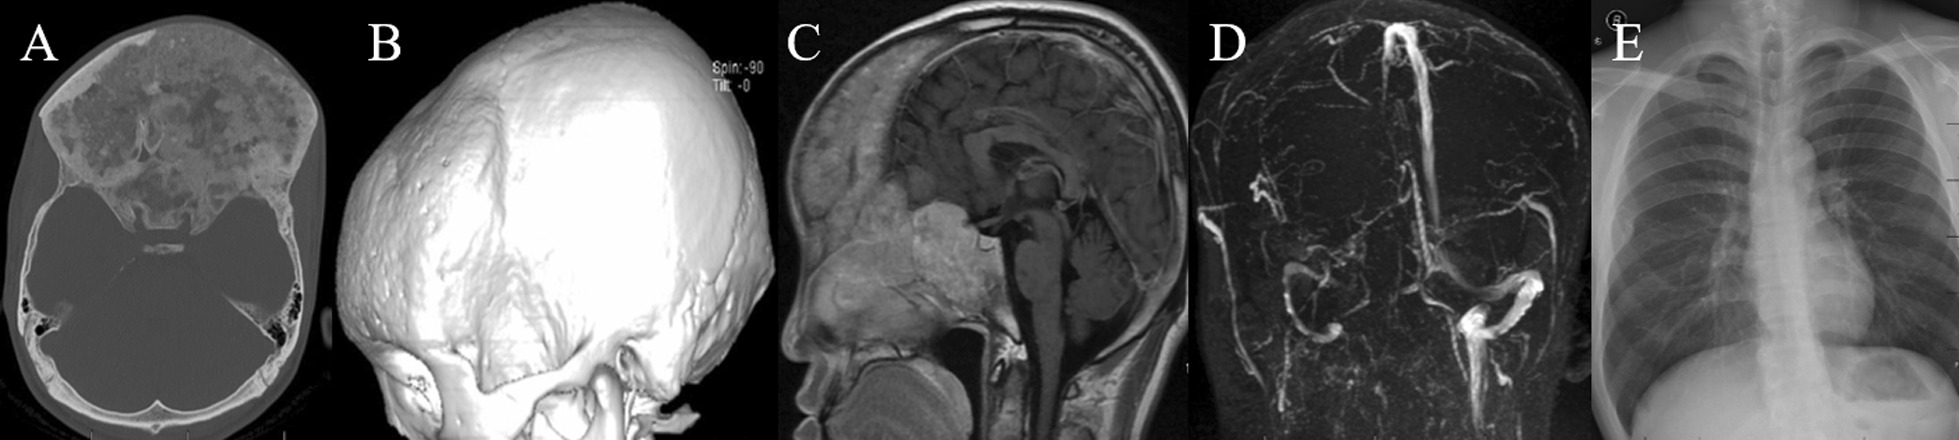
CT showing FD of Left ethmoid bone, nasal bone, sphenoid bone, bilateral orbits and frontal bones (A, B). MRI showing compression of the brain (C). MRV showing diffuse stenosis of the left venous sinuses (D). Chest X ray showing FD of the right 7th rib (E)
